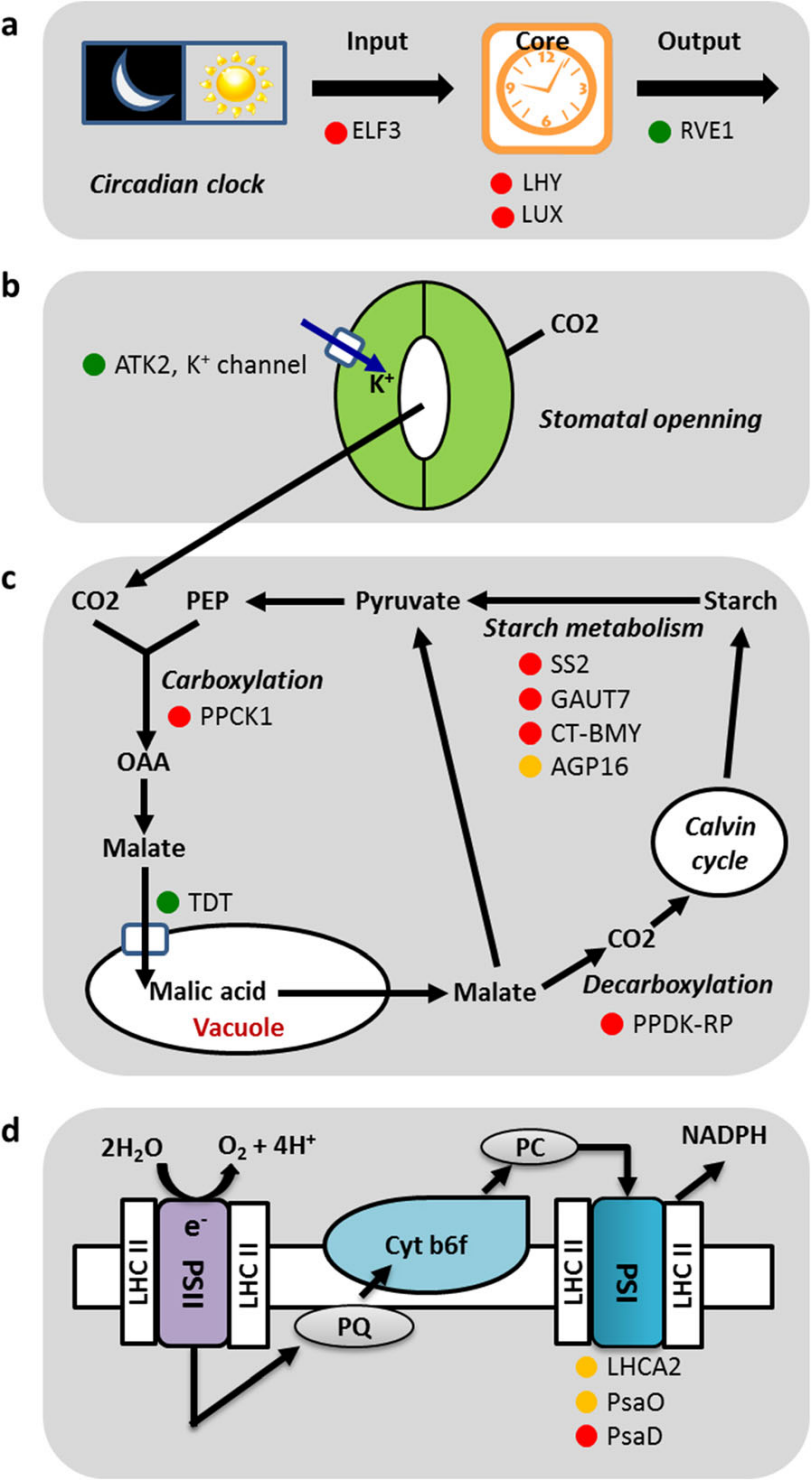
Functionally annotated *Agave* genes showing positive selection and diel rewiring of expression pattern relative to C_3_ plants. a Genes involved in circadian clock. A gene involved in stomatal opening. c Genes involved in carboxylation, malate transport, decarboxylation, and starch/sugar metabolism. d Genes involved in photosynthetic electron transport chain. Red circles indicate positive selection. Green circles indicate morning-to-night shift in peak gene expression. Yellow circles indicate afternoon-to-night shift in peak gene expression. AGP16, Arabinogalactan protein 16; AKT2, Arabidopsis Shaker family K^+^ channels 2/3; CT-BMY, Chloroplast Beta-Amylase; ELF3, Early Flowering 3; GAUT7, Galacturonosyltransferase 7; LHY, Late Elongated Hypocotyl; LUX, Phytoclock 1; PPCK1, Phosphoenlpyruvate Carboxylase Kinase 1; PPDK-RP, Pyruvate orthophosphate dikinase regulatory protein; SS2, Starch Synthase 2; TDT, Tonoplast Dicarboxylate Transporter
